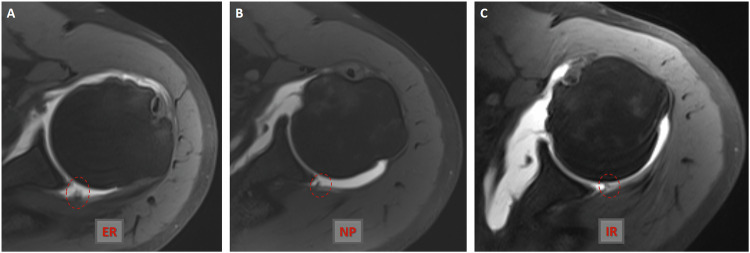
Axial fat-suppressed TSE T1-weighted MR arthrograms (A–C) obtained at the same level show a true synovial fold anomaly (circle) of the posterior shoulder joint capsule in the external rotation, neutral position, and internal rotation of the ipsilateral arm. ER, external rotation; IR, internal rotation; NP, neutral position
